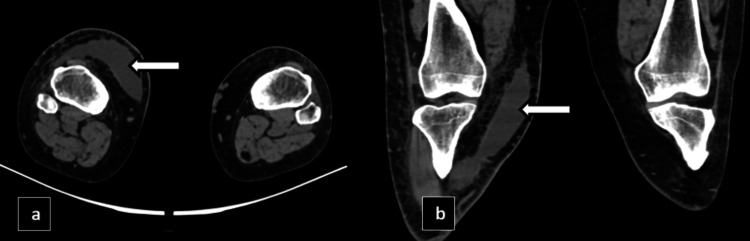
CT axial bilateral knee joint (a) and CT coronal bilateral knee joint (b) showing a fairly defined hypodense fluid collection of volume ~30-40 cc located between the subcutaneous and intermuscular planes represented by white arrows.
